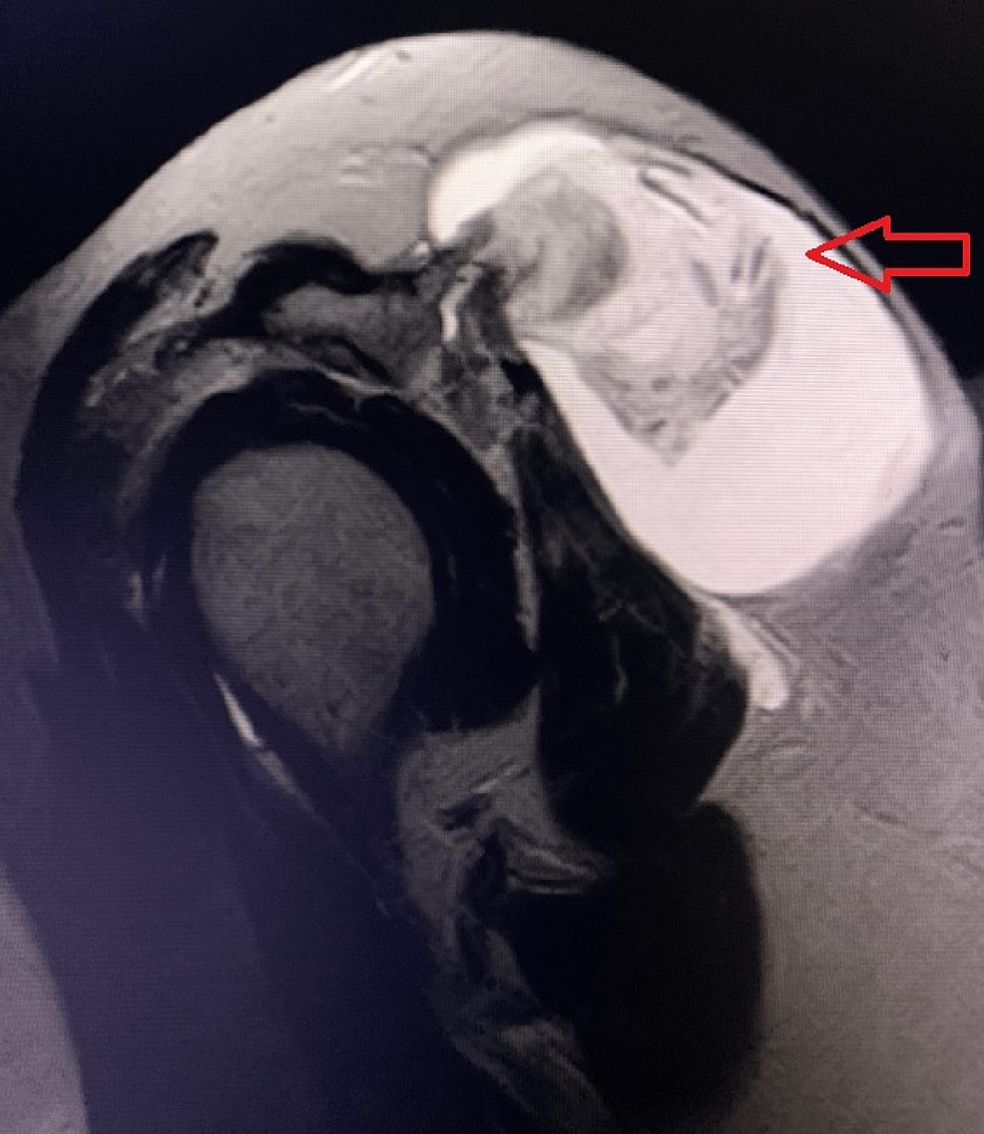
Magnetic resonance image of the left shoulder showing a cyst communicating with the glenohumeral joint fluid via a defect of the acromioclavicular joint (red arrow)
